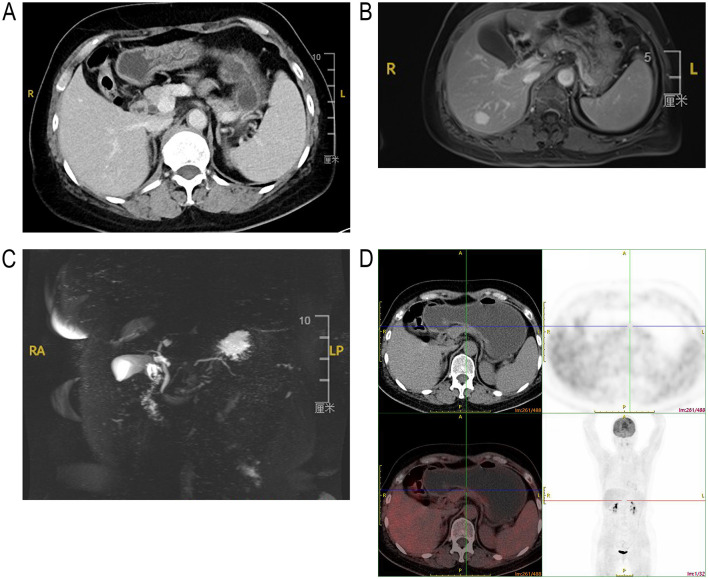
Preoperative imaging findings of pancreatic lesions. (A) CT revealed a solid lesion in the pancreatic body-neck junction, with ill-defined margins and no evidence of calcification or cystic degeneration. (B) Heterogeneous progressive mild enhancement was noted across delayed phases, with the lesion demonstrating higher signal intensity than the adjacent normal pancreatic parenchyma. (C) Magnetic resonance cholangiopancreatography (MRCP) revealing interruption of the pancreatic duct at the lesion site with mild dilation of the distal pancreatic duct. (D) PET/CT demonstrating no increased fluorodeoxyglucose (FDG) metabolism and no enhanced radioactive tracer uptake in the lesion.
